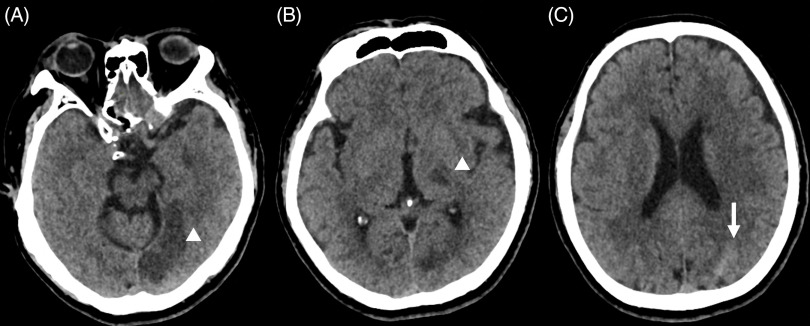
Plain cranial CT scan showing. (A) Left temporal lobe infarct (white arrowhead). (B) Left thalamic infarct (white arrowhead). (C) Subarachnoid hemorrhage at the left posterior parietal area (white arrow).
